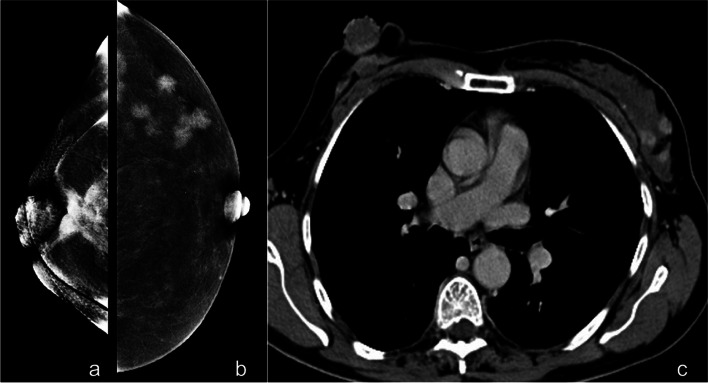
Patient with locally advanced cancer of the right breast (a) and multicentric contralateral lesions well recognized on CEM after CE-CT (b). Thoracic CE-CT scan of thorax showing bilateral breast disease (c). *CE-CT*, Contrast-enhanced computed tomography; *CEM*, Contrast-enhanced mammography
